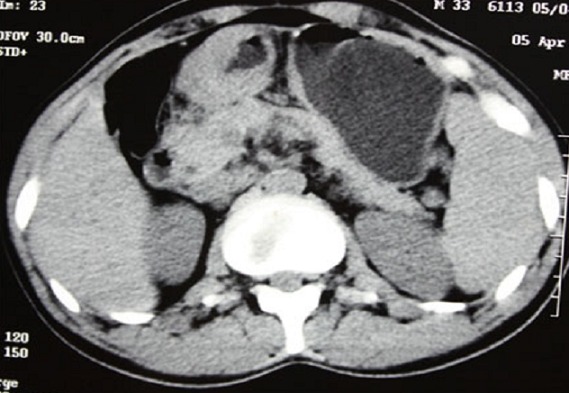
Adenocarcinoma stomach -CT scan of abdomen showing gross thickening of pylorus with regional lymphadenopathy suggestive of advanced gastric malignancy
